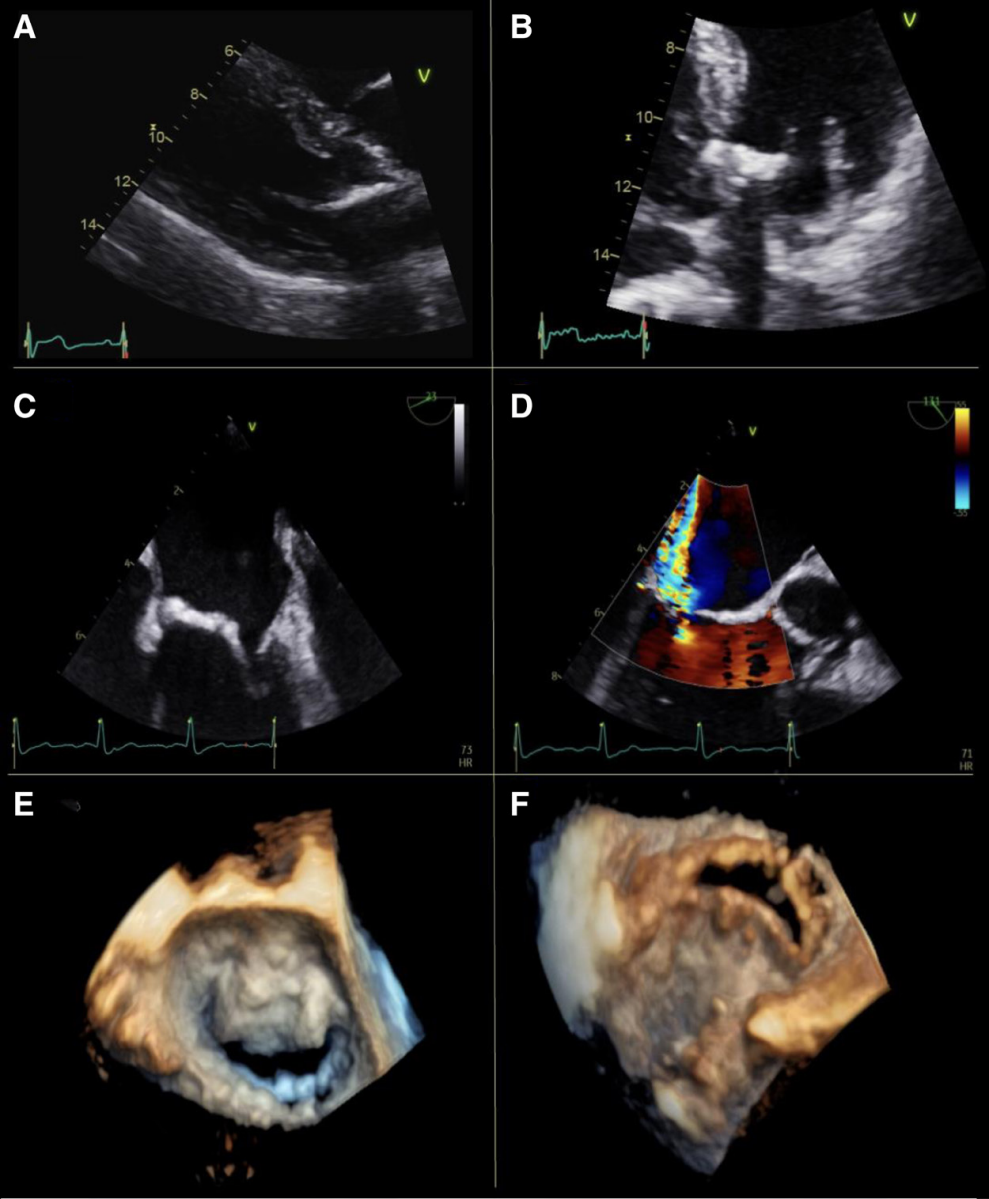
Radiotherapy-induced mitral valve changes in a 60-year-old female treated with mantle field radiotherapy for lymphoma aged 24 years. Transthoracic and transesophageal echocardiographic images A through C, Marked thickening and calcification of the basal anterior mitral valve leaflet and aorto-mitral curtain (D): posterior jet of mitral regurgitation (E and F): 4-dimensional models of the mitral valve, demonstrating relative sparing of the leaflet tips and commissures. E represents surgical view and F represents view from left ventricle.
